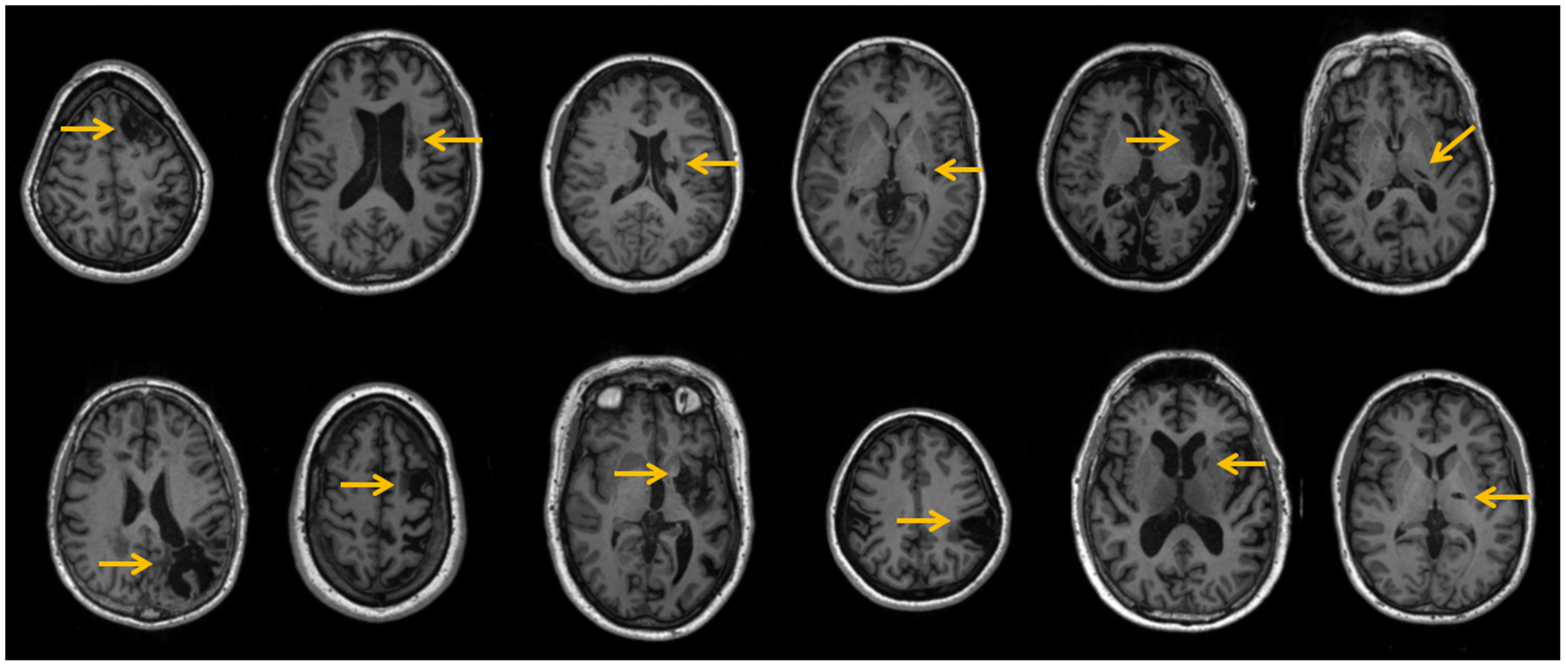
Patients' stroke lesions (yellow arrows) on T1-weighted anatomical slices.
